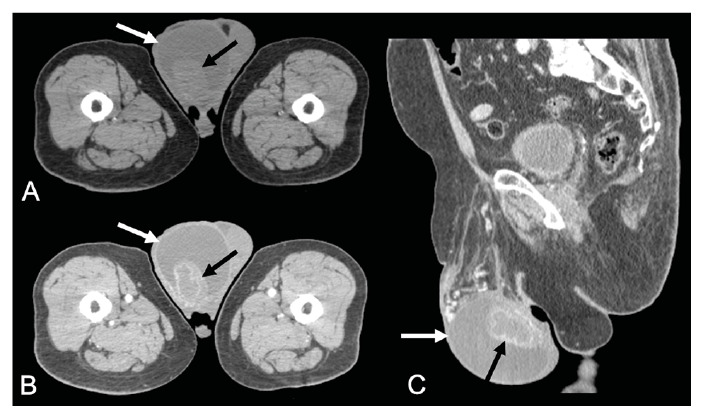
Pelvic computed tomography in the axial (A and B) and sagittal (C) planes, before (A) and after (B and C) intravenous contrast-injection showing a large hydrocele in the right scrotum (white arrows), associated with irregular right testicle and epididymis, with peripheral contrast-enhancement, and a central necrotic portion (black arrows).
